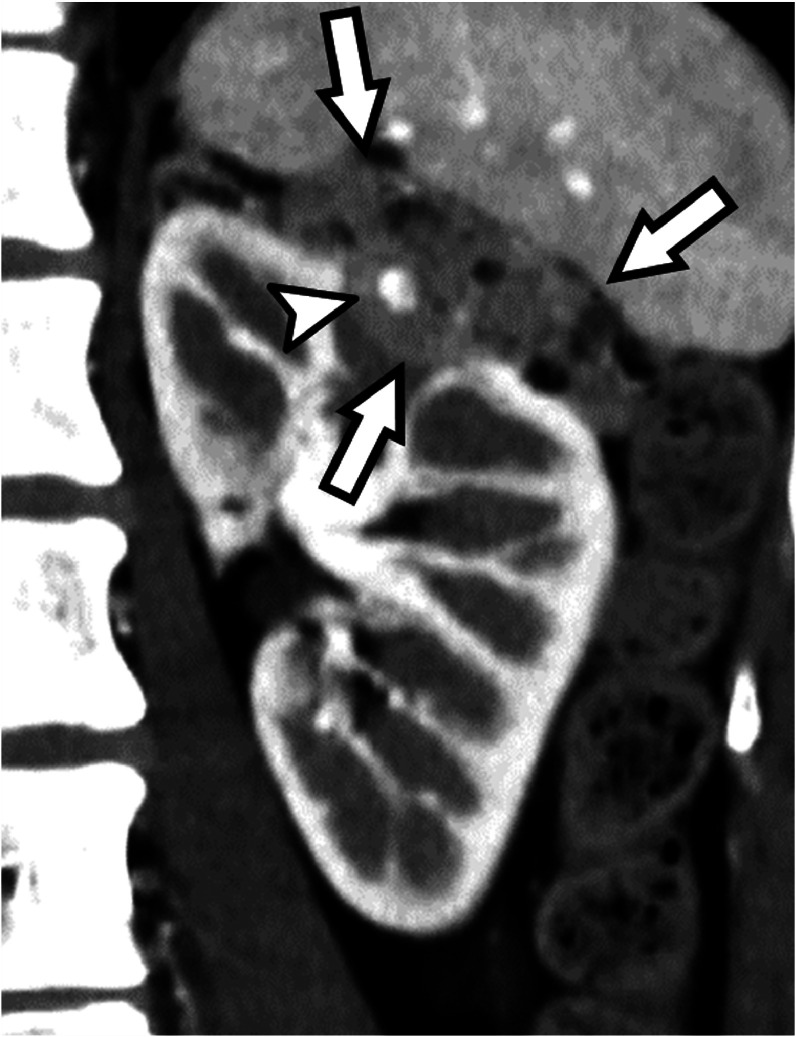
Pre-embolization contrast-enhanced computed tomography image shows a renal angiomyolipoma (arrows) with a maximum diameter of 56 mm in the left kidney and an internal aneurysm (arrowheads).
